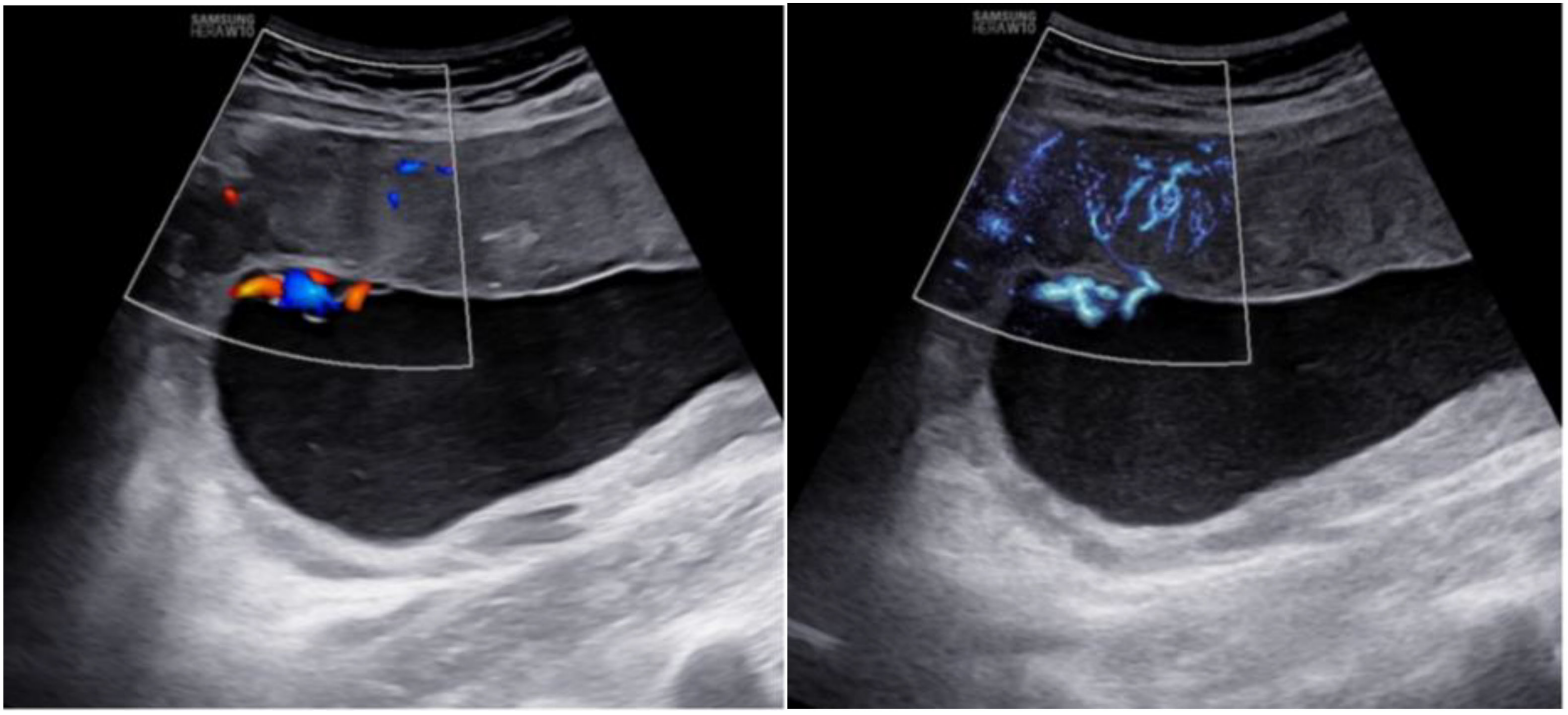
Placental imaging by routine Doppler ultrasound (left) and MV-Flow (right).
